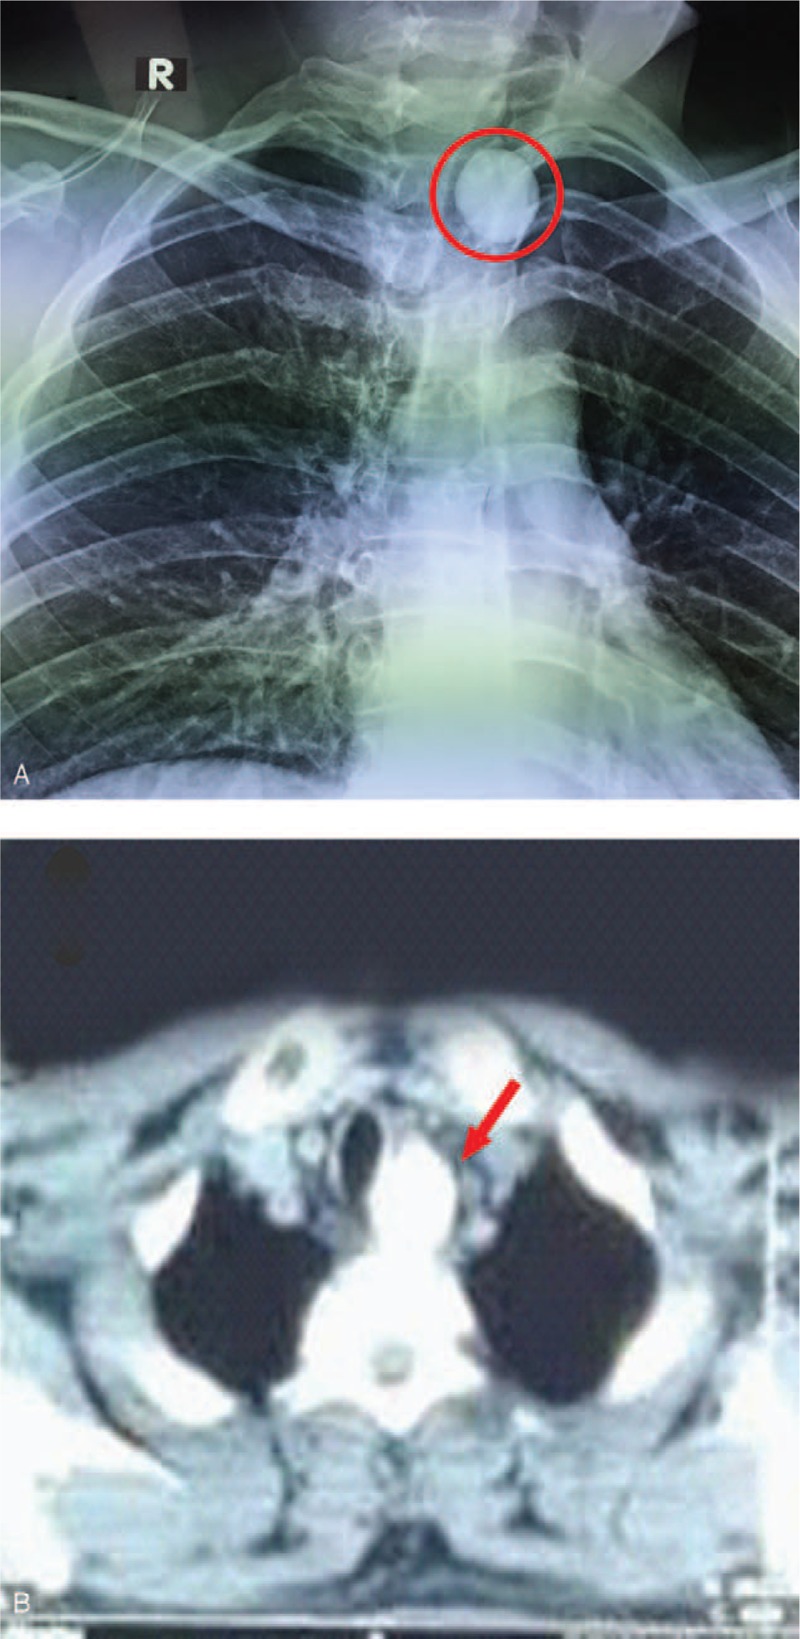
(A) Anteroposterior plain film X-ray. The red circle indicates the FB. (B) Chest CT scan. The red arrow indicates the FB.
